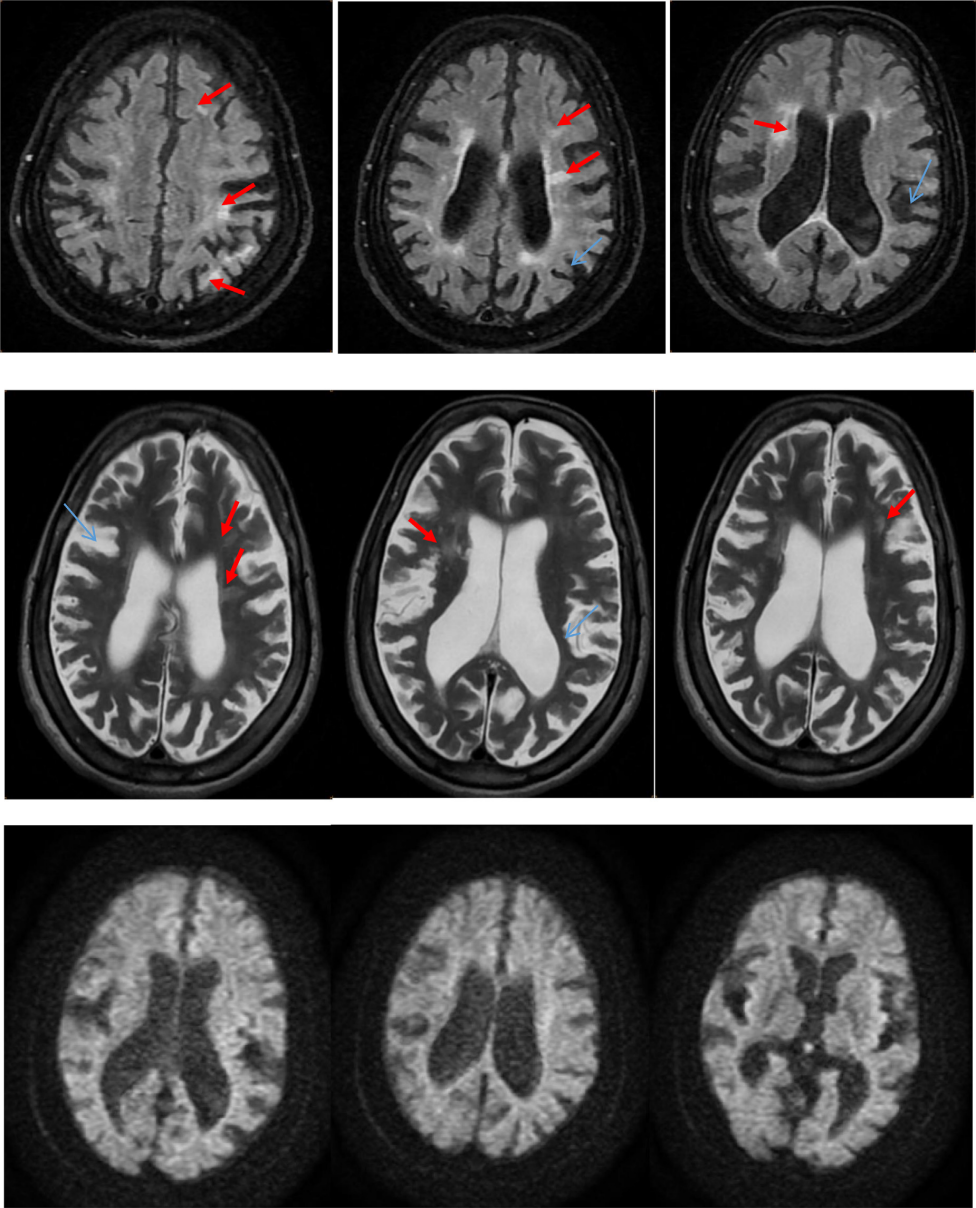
Axial MRI FLAIR, T2, and diffusion sequences showing multiple hyperintense areas in the periventricular deep white matter and bilateral frontal and parietal territories (red arrows), without diffusion restriction, and associated with cortical atrophy (blue arrows).
